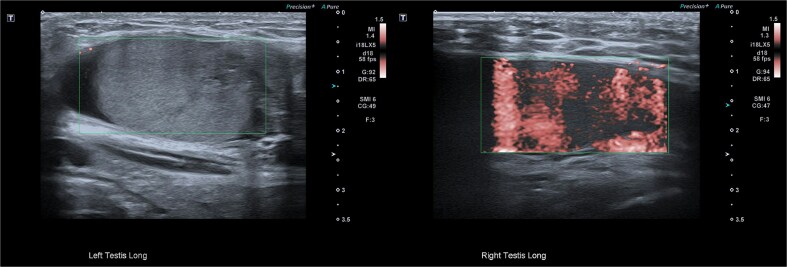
Pre-operative ultrasonographic image demonstrating left and right intracanalicular testis. The left testis has absent colour Doppler flow and heterogeneous echotexture concerning for compromised perfusion.
